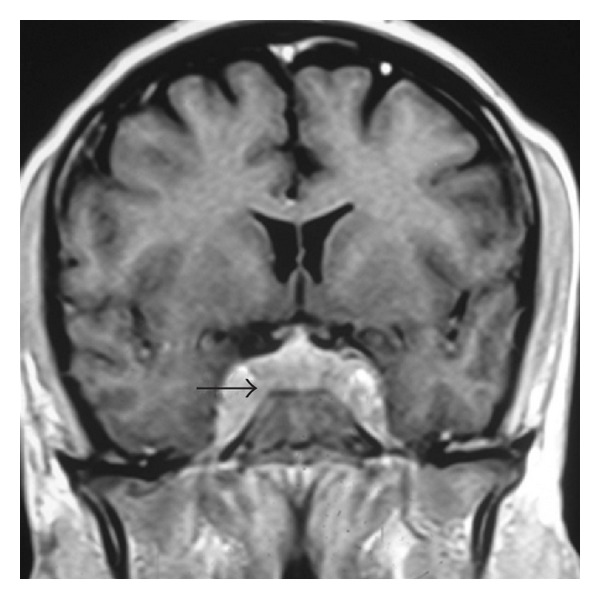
Coronal T1-weighted contrast image shows enhancing lesion in the cavernous sinus bilaterally with sphenoid sinus fullness (black arrow).
